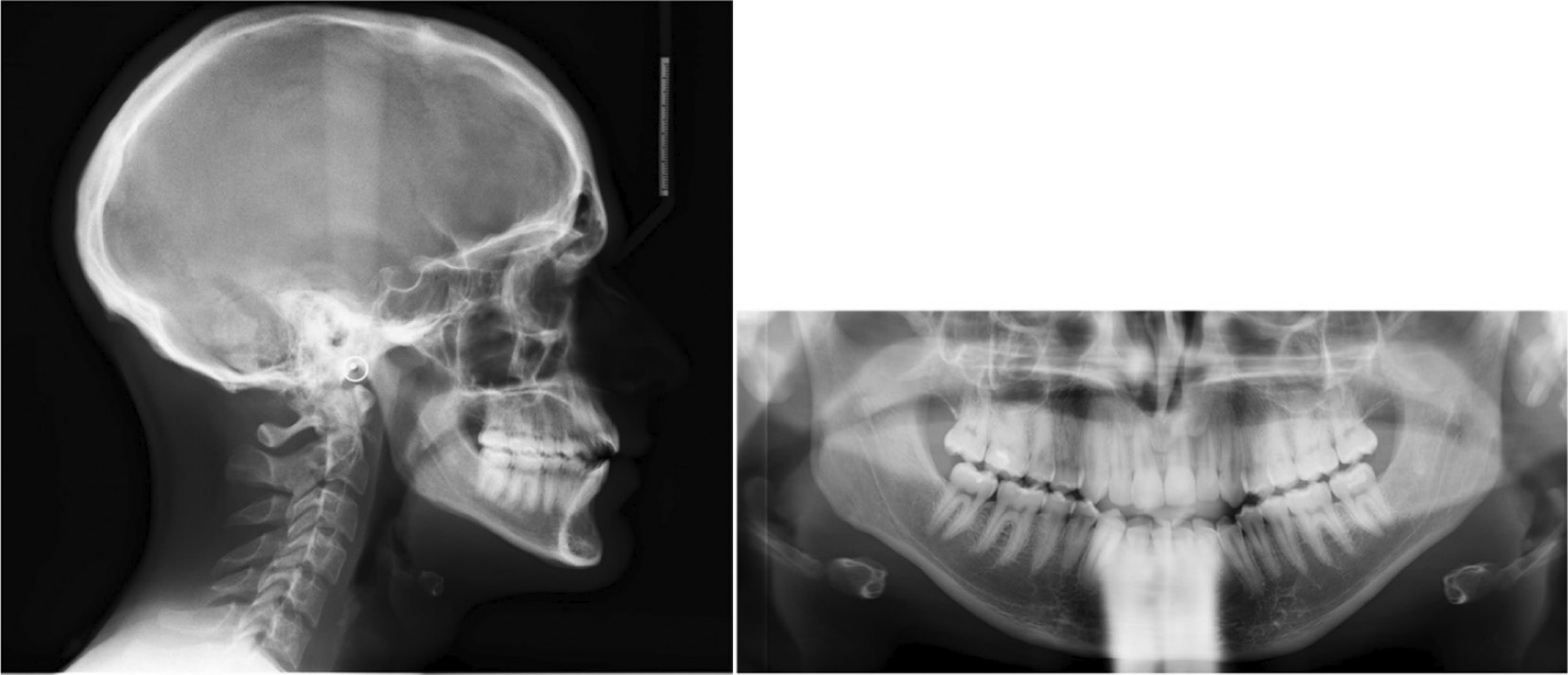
Pre‐treatment radiographs
